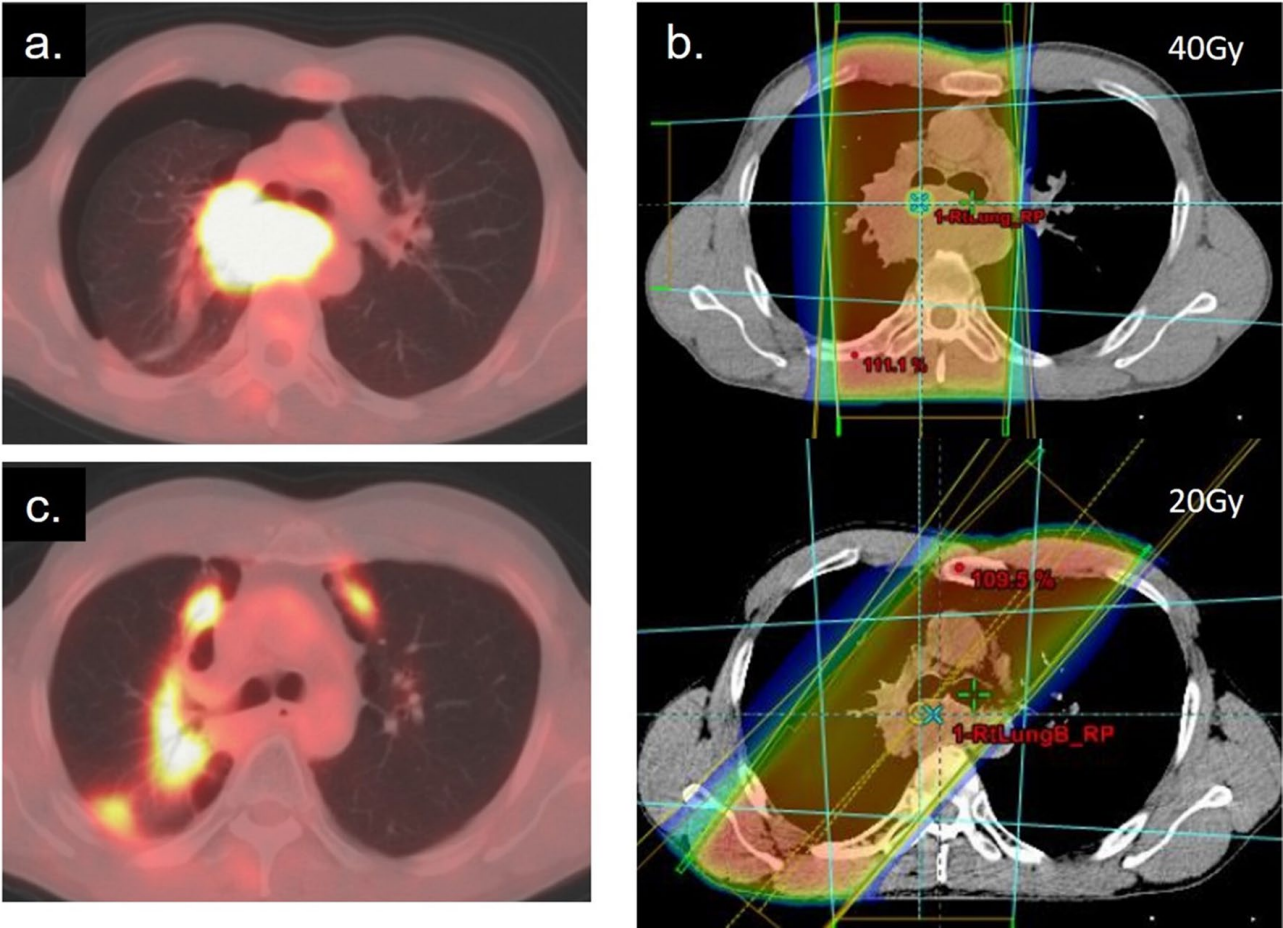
Axial images of a case of radiation pneumonitis that occurred in a patient with esophageal cancer. a FDG–PET/CT before treatment, b image of the irradiation field with two radiation direction (1. 40 Gy (upper) and 2. 20 Gy (lower)), c FDG–PET/CT 11 months after radiotherapy
